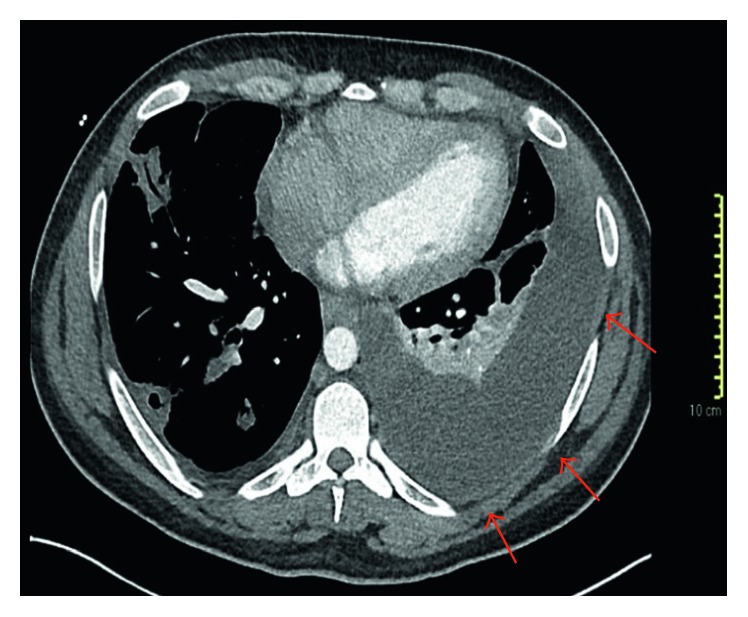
Arterial-phase axial computed tomographs of the lower thorax demonstrating a moderate left pleural effusion with associated compressive atelectasis. Peripheral cavitary pulmonary lesions and partially visualized tricuspid valve (with known vegetation) are shown.
